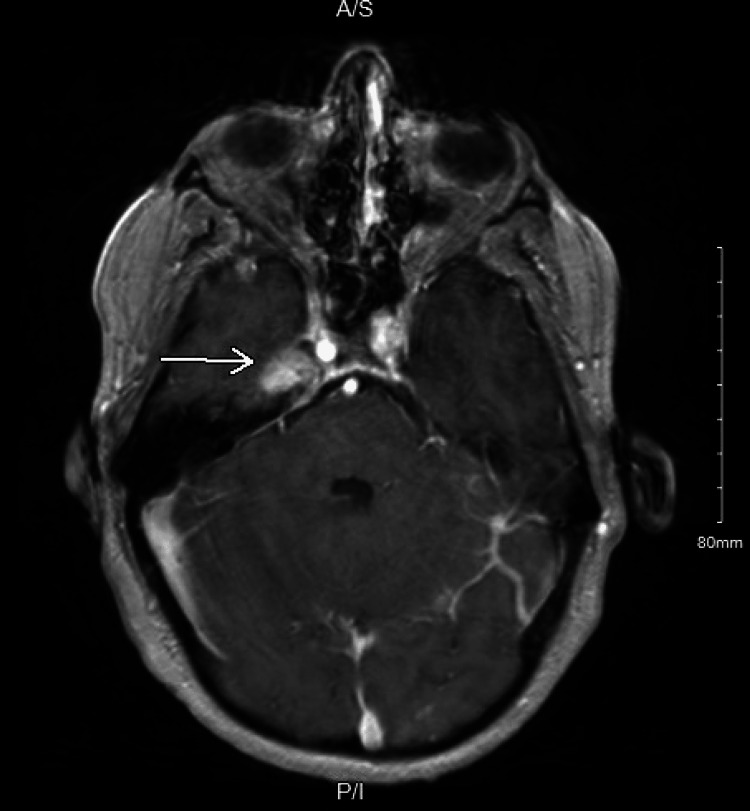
MRI brain revealing 1.4 cm mass at the posterior margin of Meckel’s cave (arrow)
